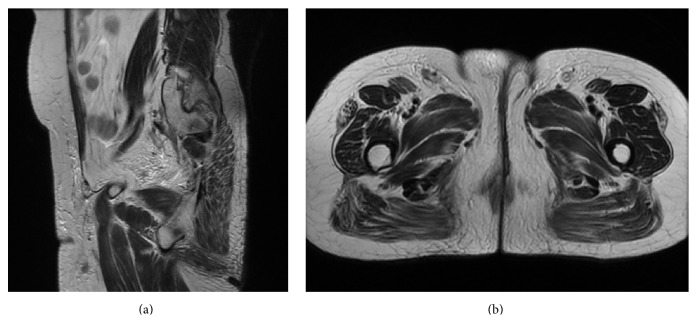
((a) and (b)) Posttreatment MR images in sagittal and axial section show complete resolution of the inguinal lymph nodes.
